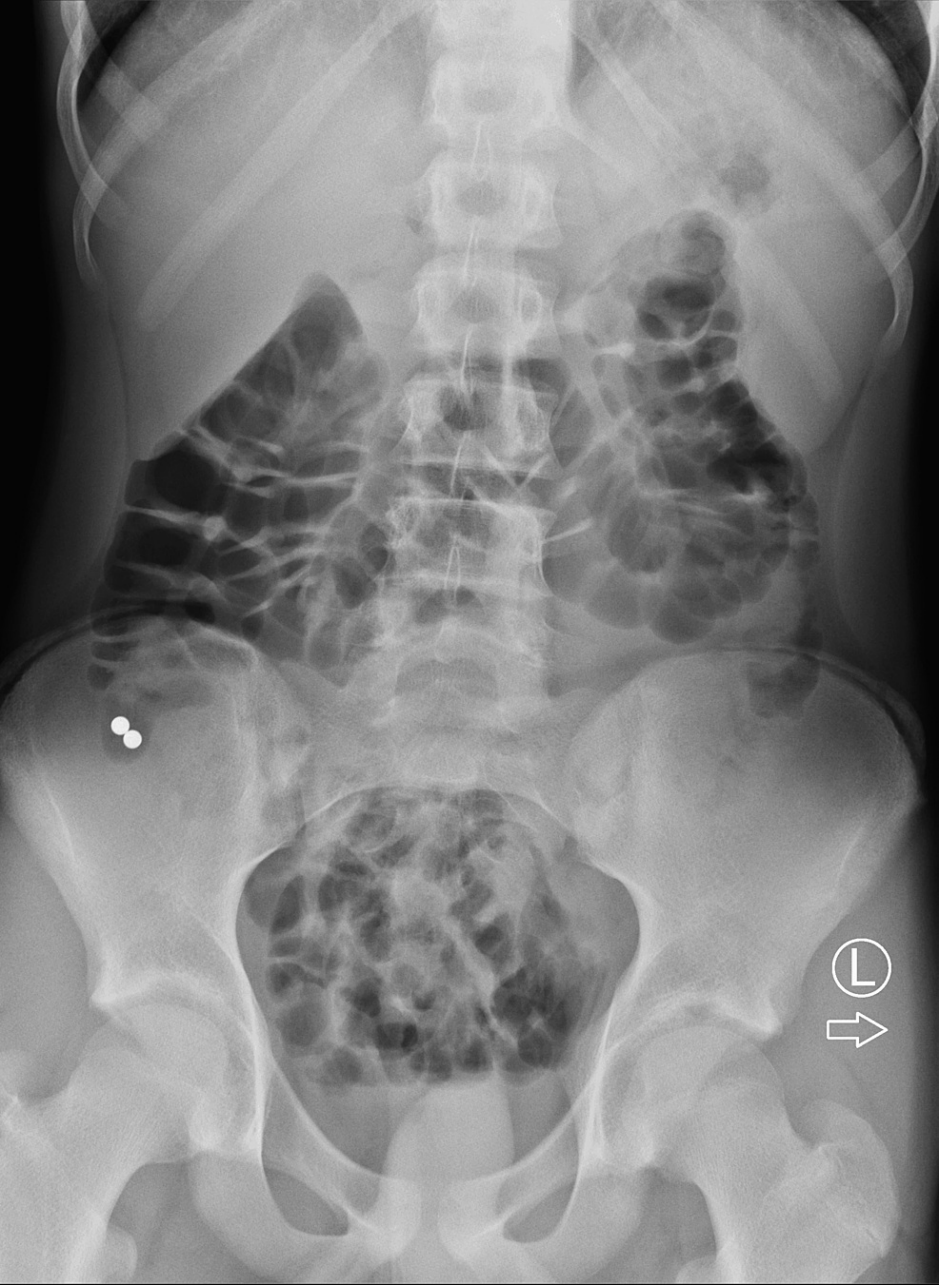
Abdominal X-ray showing two magnetic balls in the cecum.
